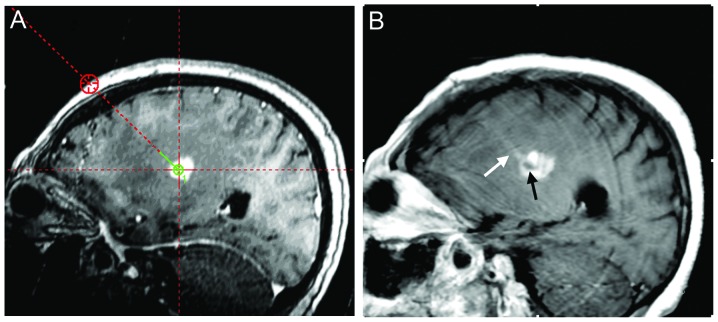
Comparision of pre-operative and intraoperative contrast-enhanced magnetic resonance imaging (MRI) images. (A) The ideal target site and a reasonable needle track were identified using the Leksell stereotaxic planning system. (B) The intraoperative MRI (iMRI) image revealing the biopsy trajectory (white arrow). Immediate iMRI identified no surgical bleeding, and the biopsy trajectory reached the target site (black arrow).
